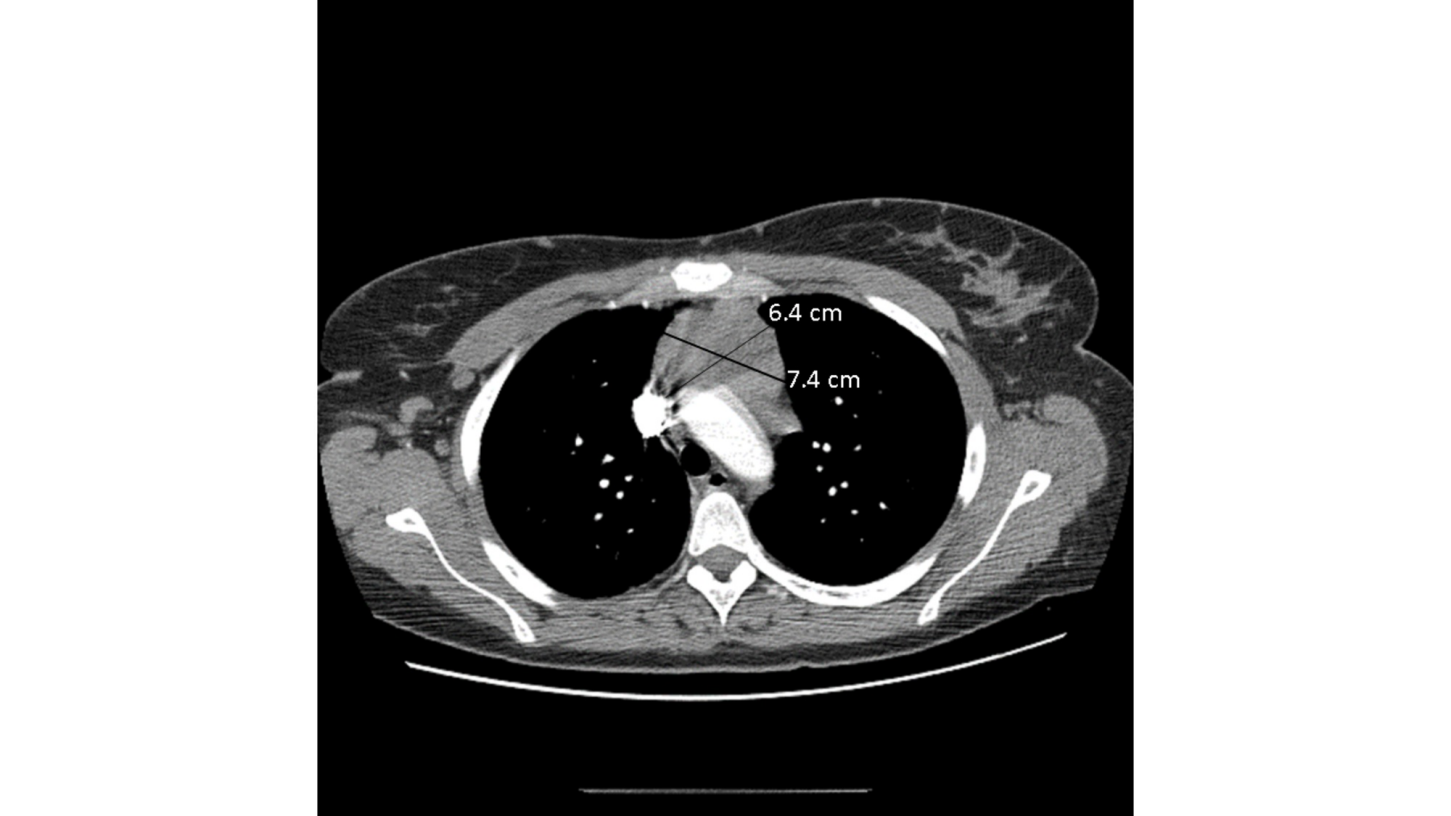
Contrasted computed tomography (CT) images of the chest showing preoperative lobular mass in the anterior mediastinum.
